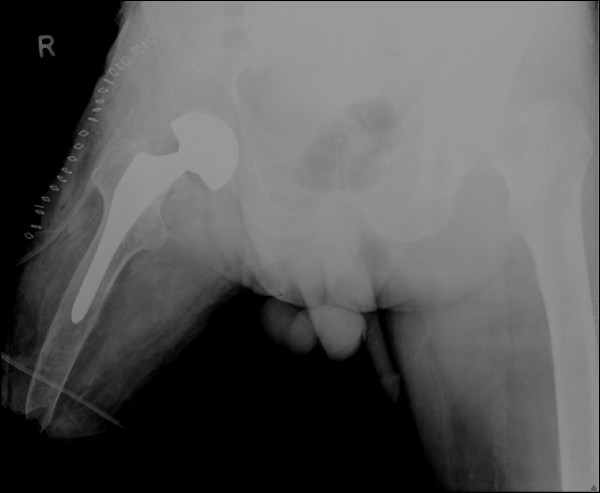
Postoperative xray with an uncemented bipolar hemiarthroplasty.
